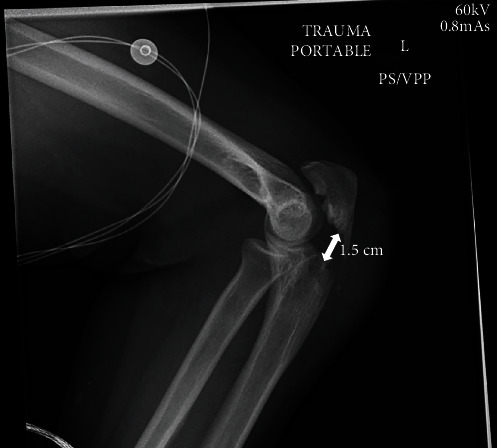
Preoperative lateral X-ray of the left elbow showing fracture of the olecranon process with displacement of 1.5 cm.
